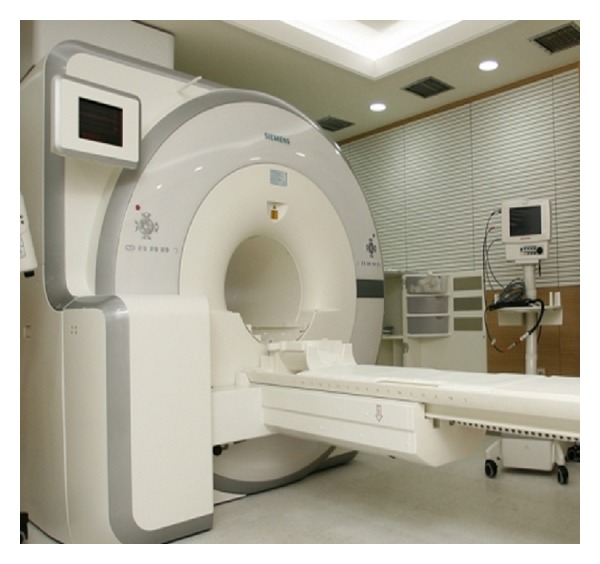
PET/MRI scanner (Biograph mMRI, 3T Siemens, Erlangen, Germany).
